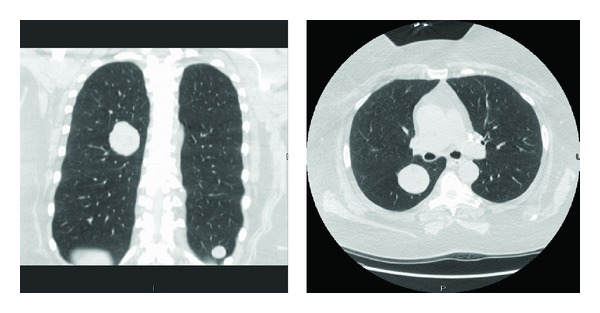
CT: well-defined noncalcified pulmonary nodules.
